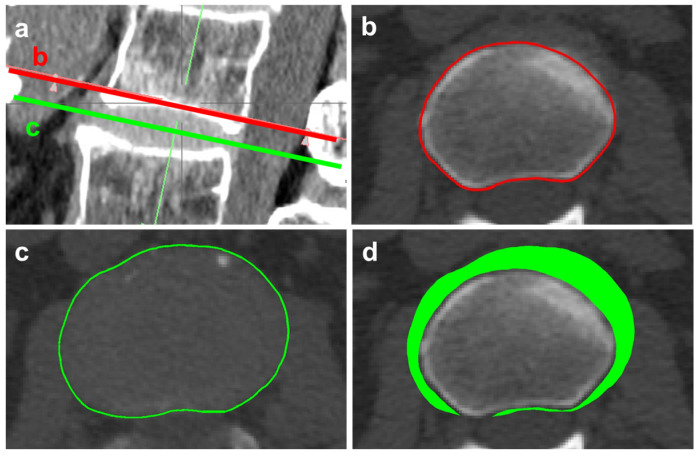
Measurement of % extended area of disc (EAD). Sagittal reconstruction image of lumbar CT (a). The cross-sectional area of endplates (endplate area) enclosed by red line was determined at the concave apex of the superior endplate of each disc level (b). The cross-sectional area of IVDs (disc area) enclosed by green line was determined at the midline between the superior and inferior endplates (c). Extended area of disc (EAD) filled in green was calculated as the subtraction of the endplate area from the disc area (d). %EAD was calculated as follows: (disc area-endplate area)/endplate area ×100.
